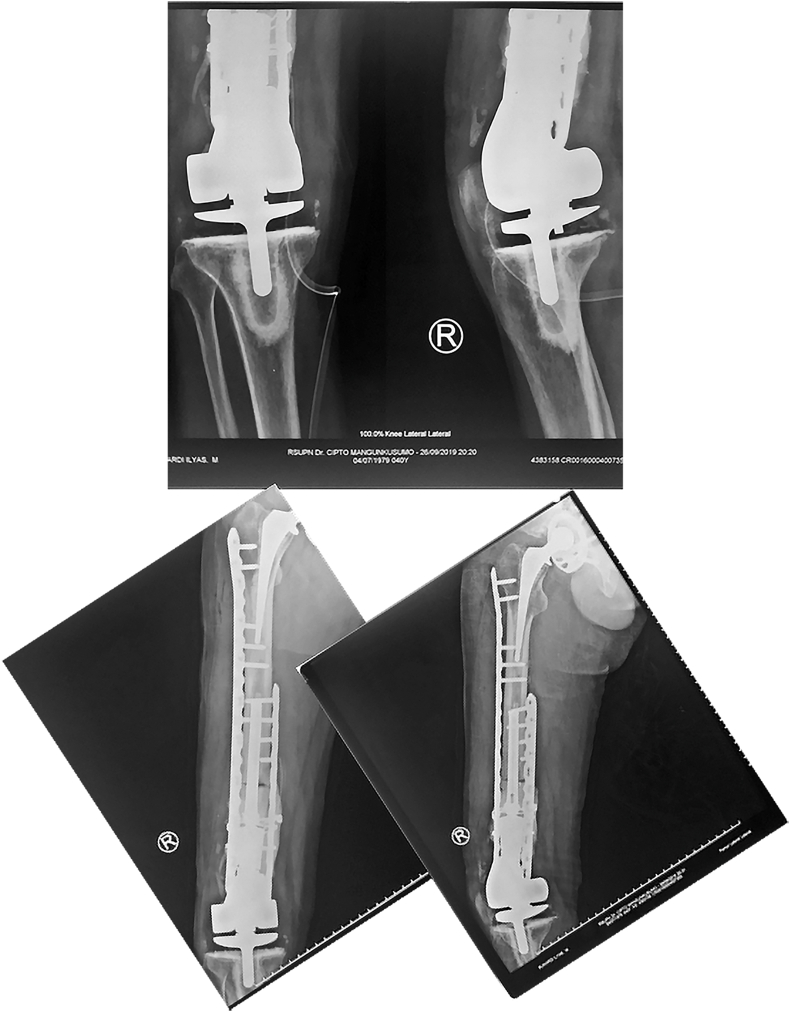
Post-operative x-ray in June 2020.
